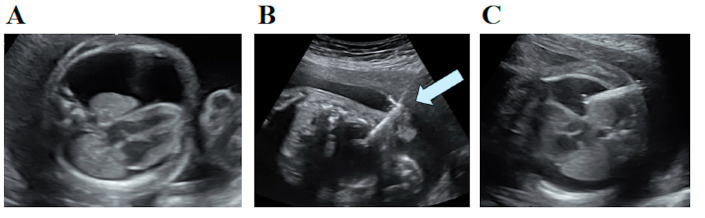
Prenatal ultrasound images (A) Bilateral hydrothorax larger on the left size detected at 21+6 weeks (B) Somatex^®^ shunt placement on left hydrothorax under ultrasound guidance (C) Control scan at 30+4 weeks.
